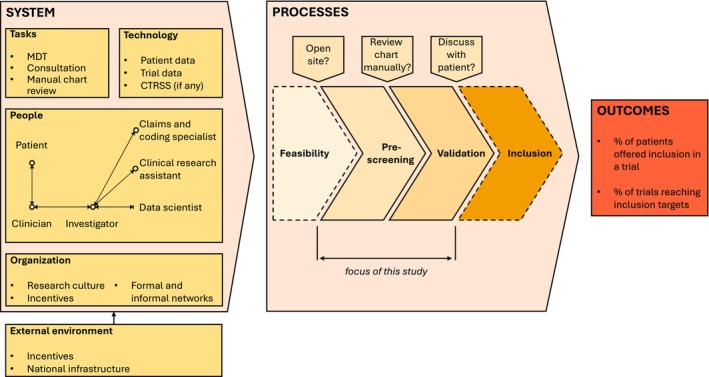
Work system for patient eligibility screening for clinical trials in oncology, mapped onto the SEIPS 2.0 framework [34]. Our study focuses on the ‘pre‐screening’ and ‘validation’ stages. We did not identify any element of the ‘internal environment’ affecting CT recruitment. CTRSS, Clinical trial recruitment support system; MDT, Multidisciplinary team meeting.
